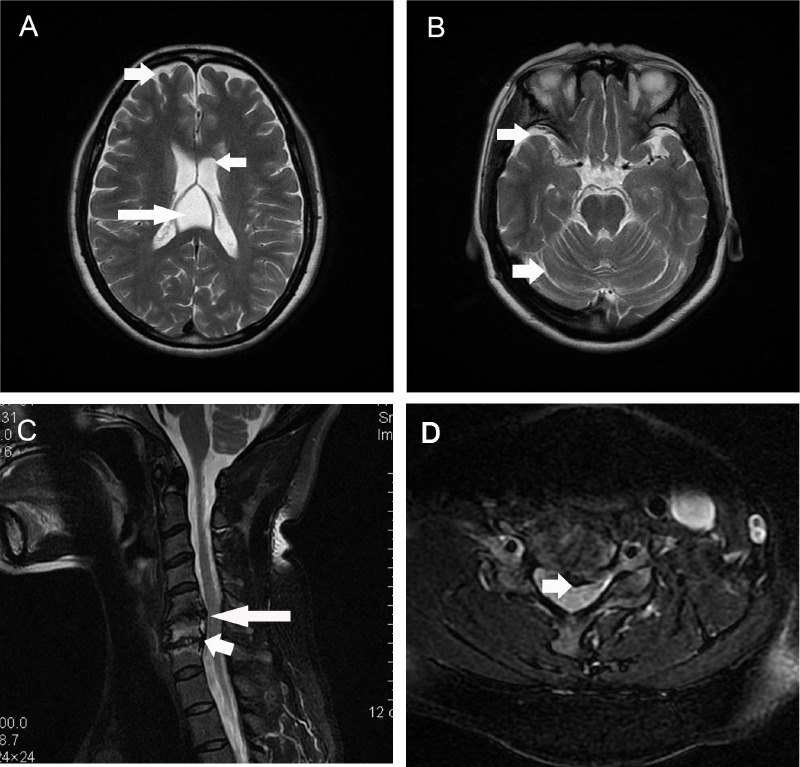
Magnetic resonance imaging (MRI) of the brain and spinal cord. Brain MRI showed existence of cavum vergae long arrow on panel (A), and relatively deep sulcuses short arrow on panels (A and B) suggesting mild diffuse brain atrophy (A, B). Intervertebral herniation in cervical 5/6 (C5/6) long arrow on panels (C and D) and C6/7 short arrow on panel (C) was detected by cervical spinal cord MRI, with mild degeneration of the associated spinal cord.
